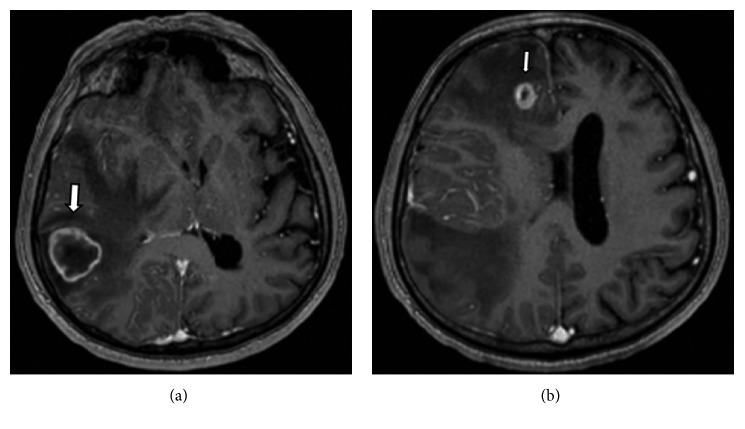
Contrast-enhanced T1-weighted images of the brain. (a) There is a ring enhancing approximately 3 cm mass (white thick arrow) in the right temporoparietal region with surrounding edema. This results in effacement of the right lateral ventricle and mild leftward midline shift. This was the dominate mass of the 3 lesions initially identified. (b) Similar smaller ring enhancing lesion (white thin arrow) in the right frontal lobe with surrounding edema.
